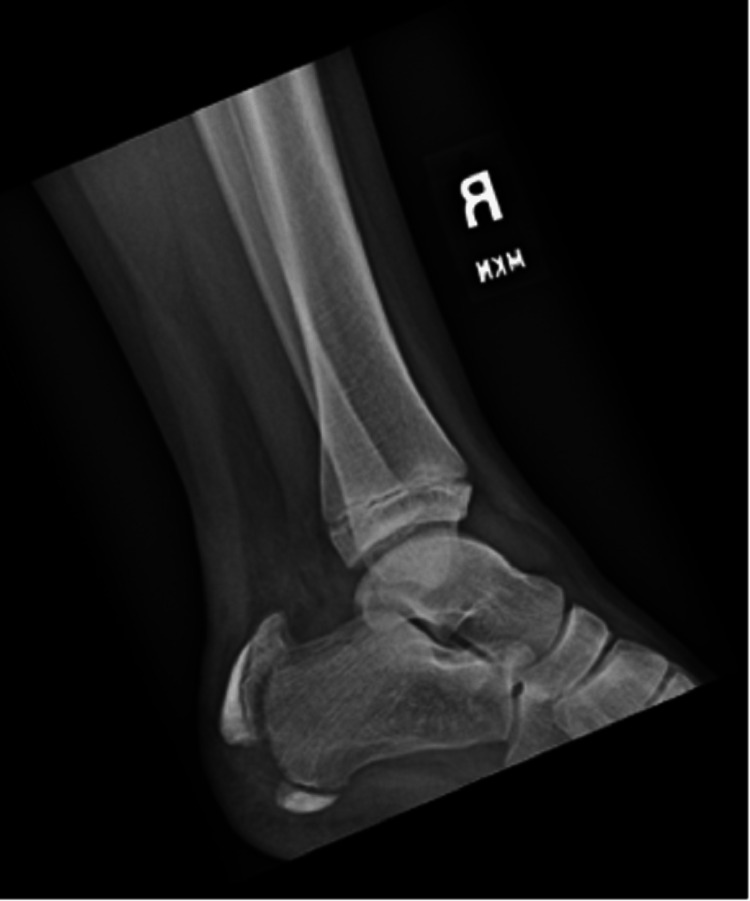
Plain Lateral radiograph of the right foot demonstrating a displaced fracture through the apophysis of the calcaneus with a cranial displacement of the proximal fracture fragment.
